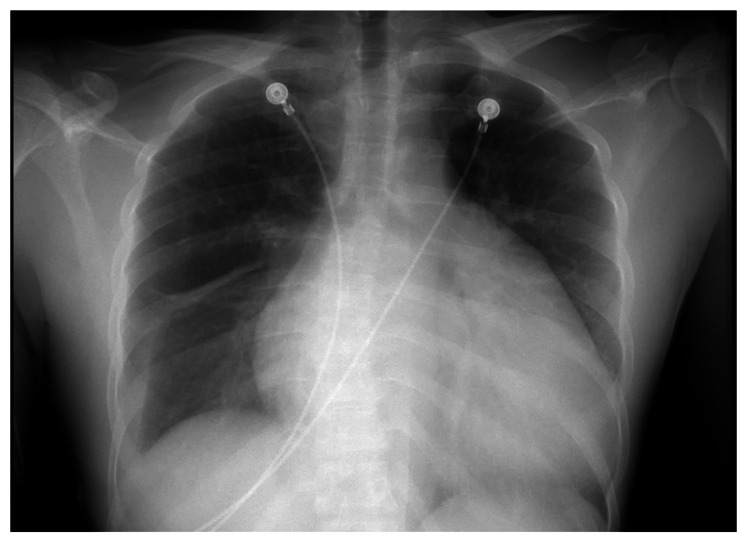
CXR showing cardiomegaly, vascular congestion, and small bilateral pleural effusion CXR: Chest X-ray
